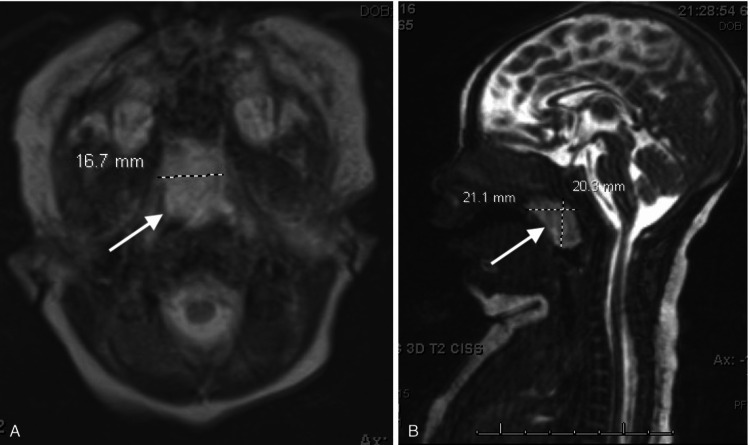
MRI of nasopharyngeal mass in axial (A) and sagittal (B) views measuring 2.1 x 1.7 x 2 cm. MRI: magnetic resonance imaging
